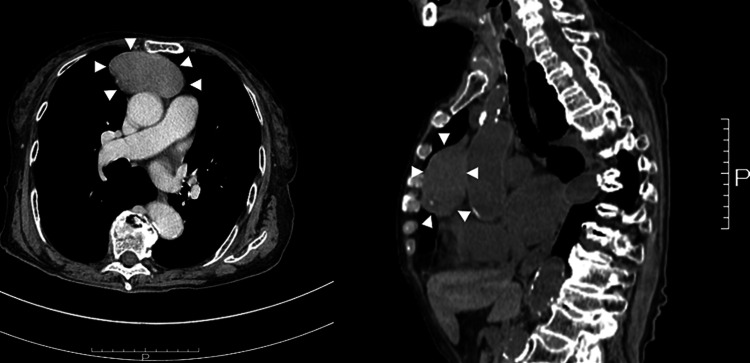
Computed tomography (CT) chest scan (left and right) showing a well-defined, 7-cm soft tissue mass in the anterior mediastinum.
